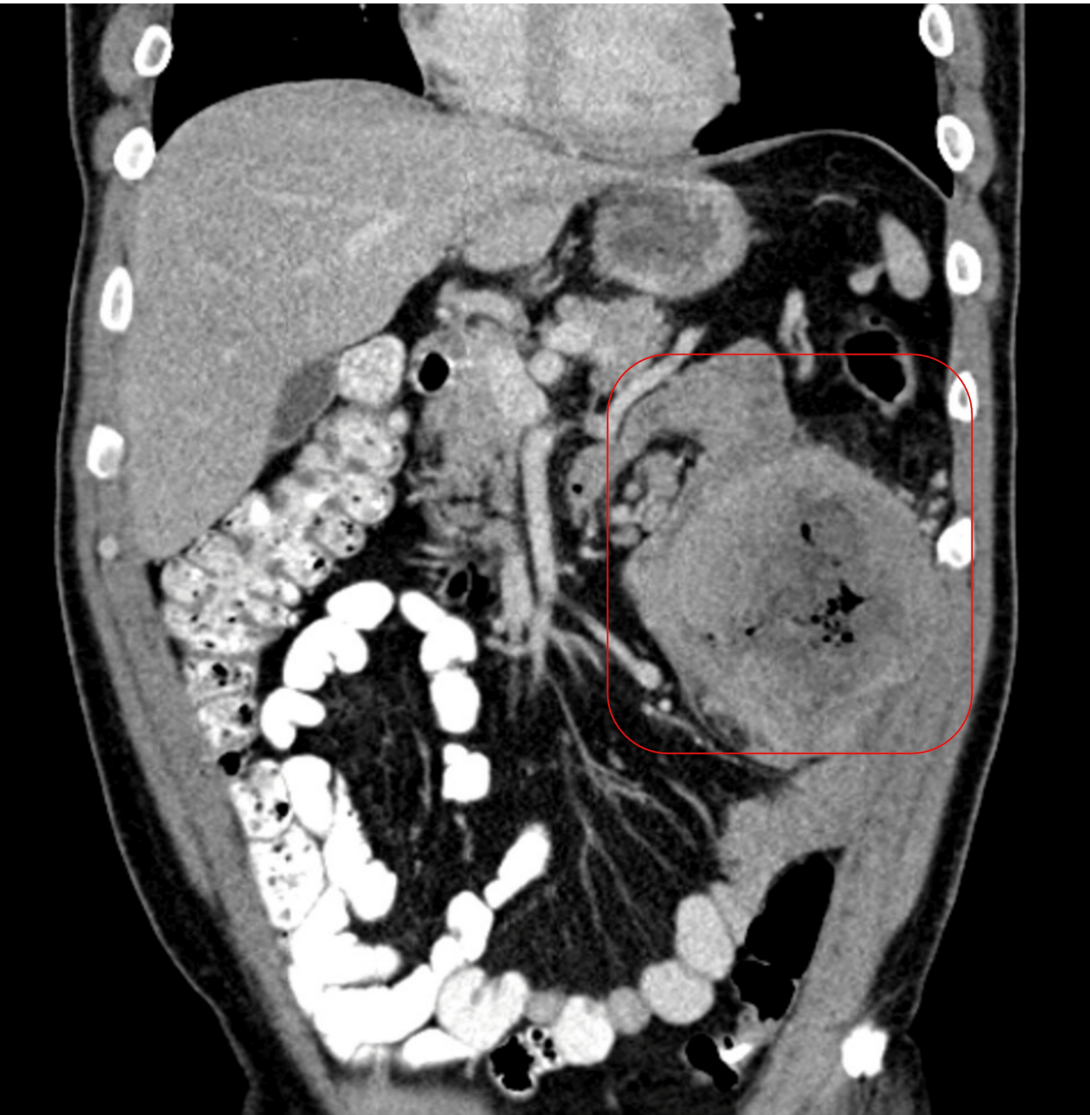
Coronal view of contrast-enhanced CT of the abdomen and pelvis showing a left-sided abdominal mass measuring 11.7 x 11.7 x 10.3 cm (red square).
